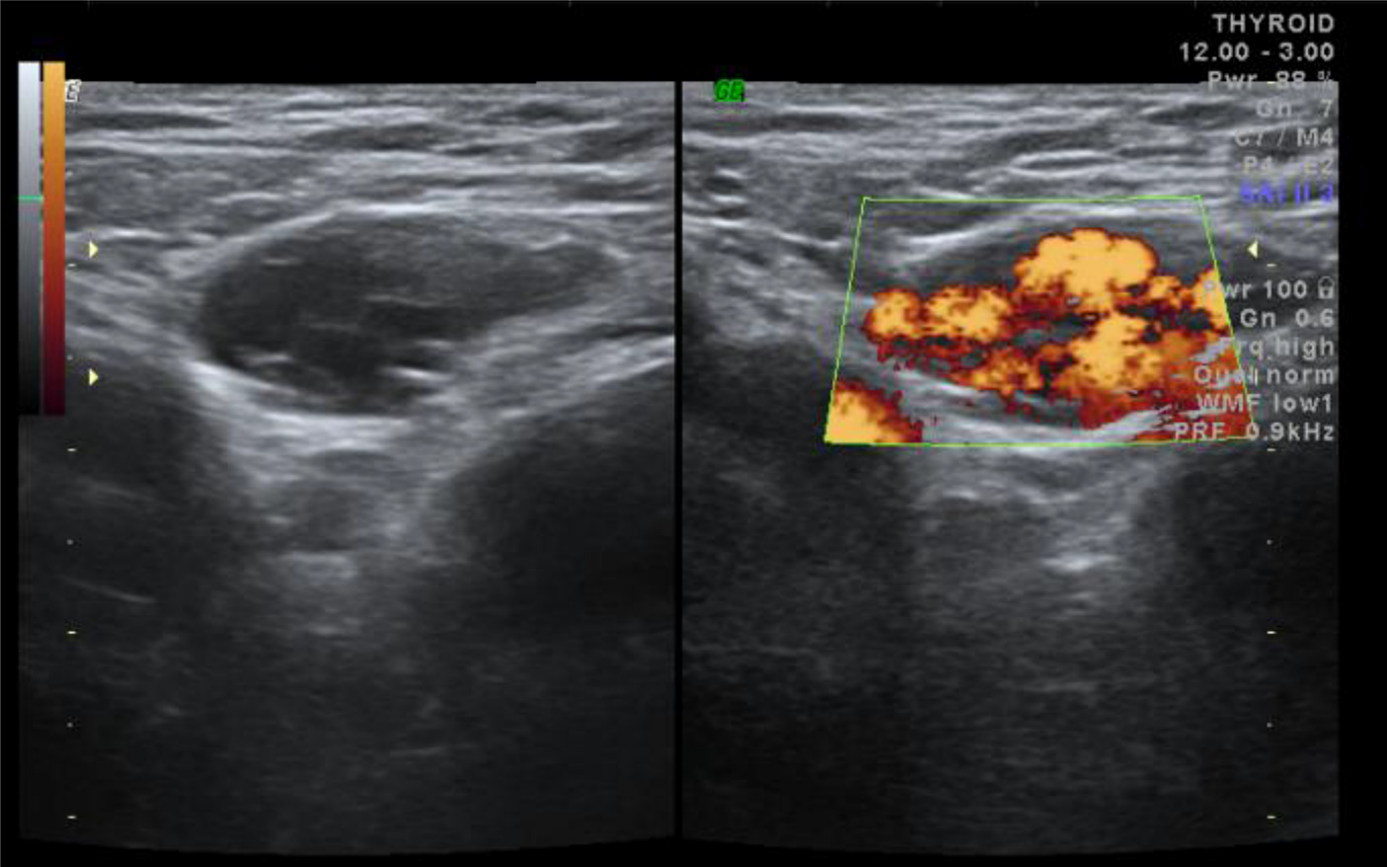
Transverse gray scale (left) and power Doppler (right) image in the right inguinal region showing vascular channels with flow signals on power Doppler.
